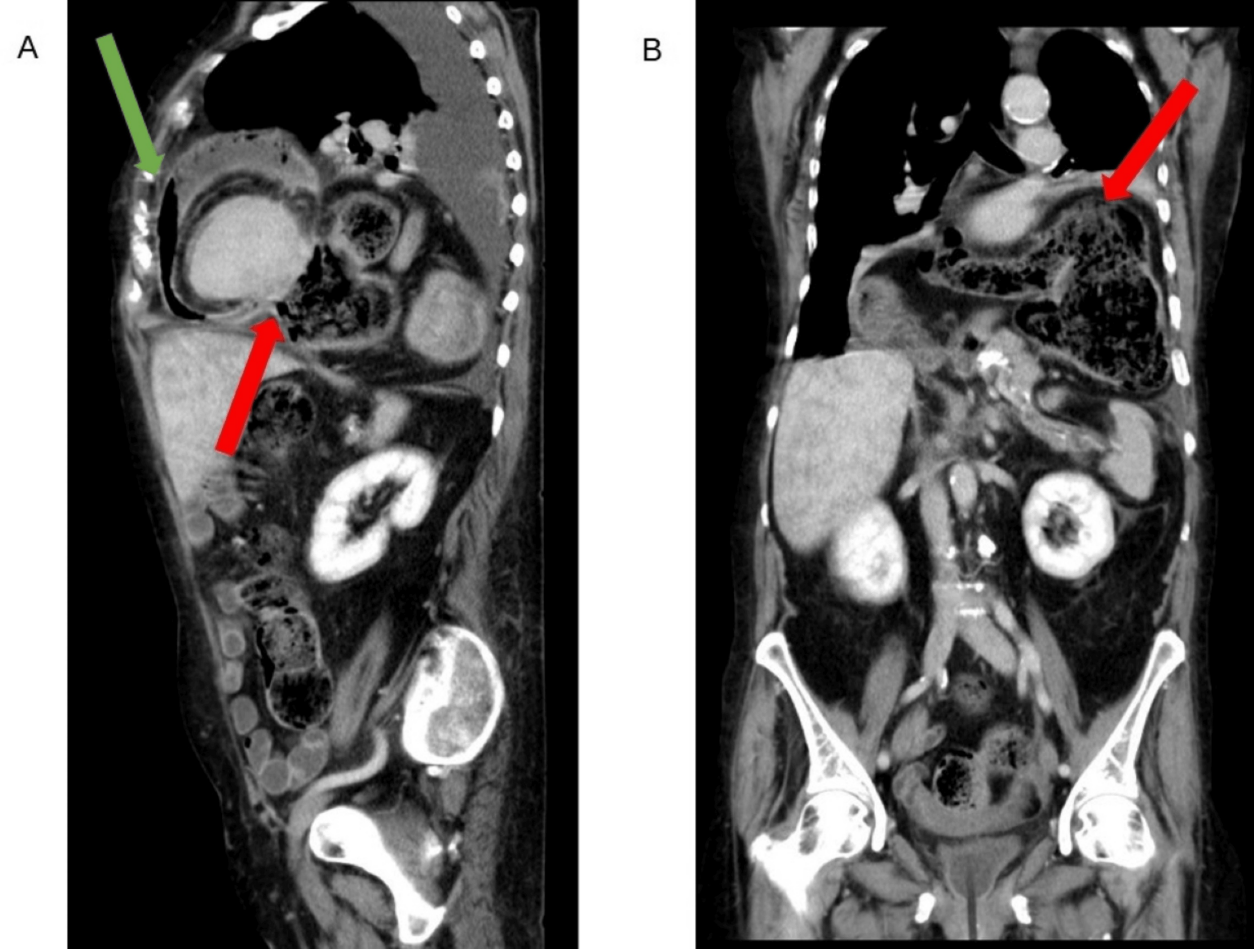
Chest and abdominal CT, venous phase, lateral and anteroposterior reconstruction (A) Lateral reconstruction: the green arrow shows the pericardial effusion with gas, and the red arrow shows the transverse colon perforation with the fistula to the pericardium; (B) anteroposterior reconstruction: the red arrow shows the giant hiatal hernia that compromised the left lung and mediastinum.
